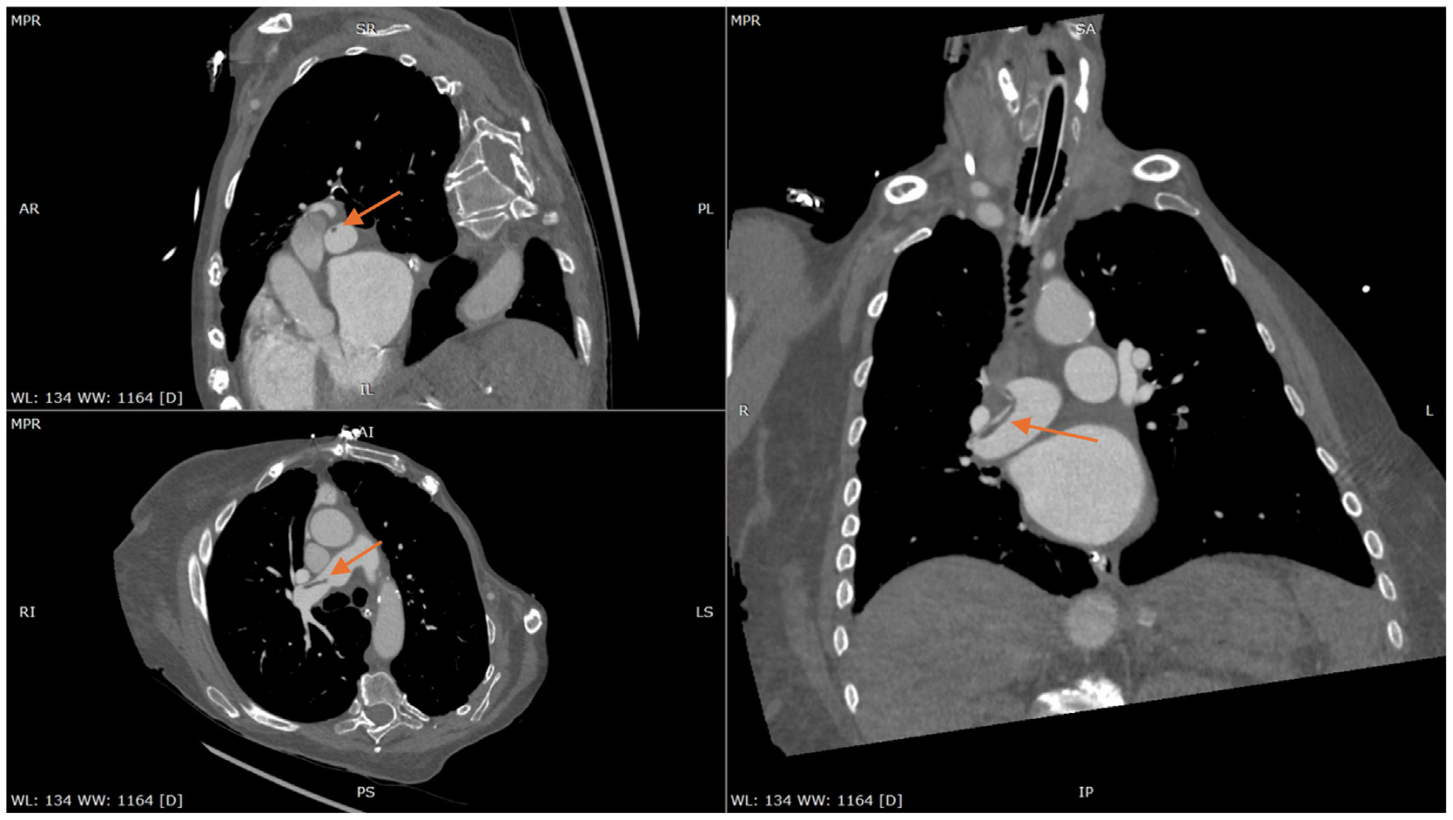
Computed tomography pulmonary angiogram (CTPA) demonstrating small pulmonary emboli (PE) presumed to be fat embolism (FE) based on the Hounsfield units (HUs), which has been rotated with three-dimensional multiplanar reconstruction to better visualize the FE, which is marked with *orange arrows*.
